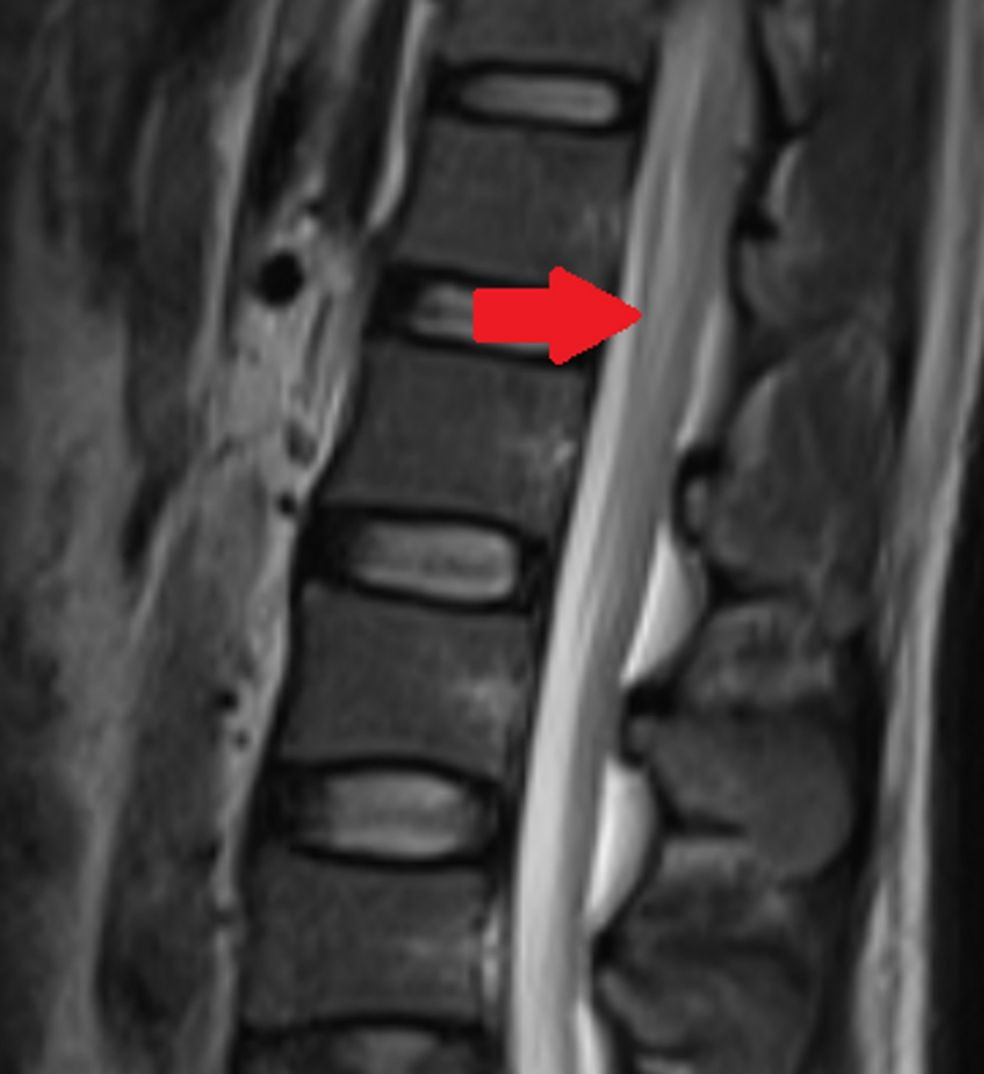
MRI spine showing surface enhancement over cauda equina nerve roots.
